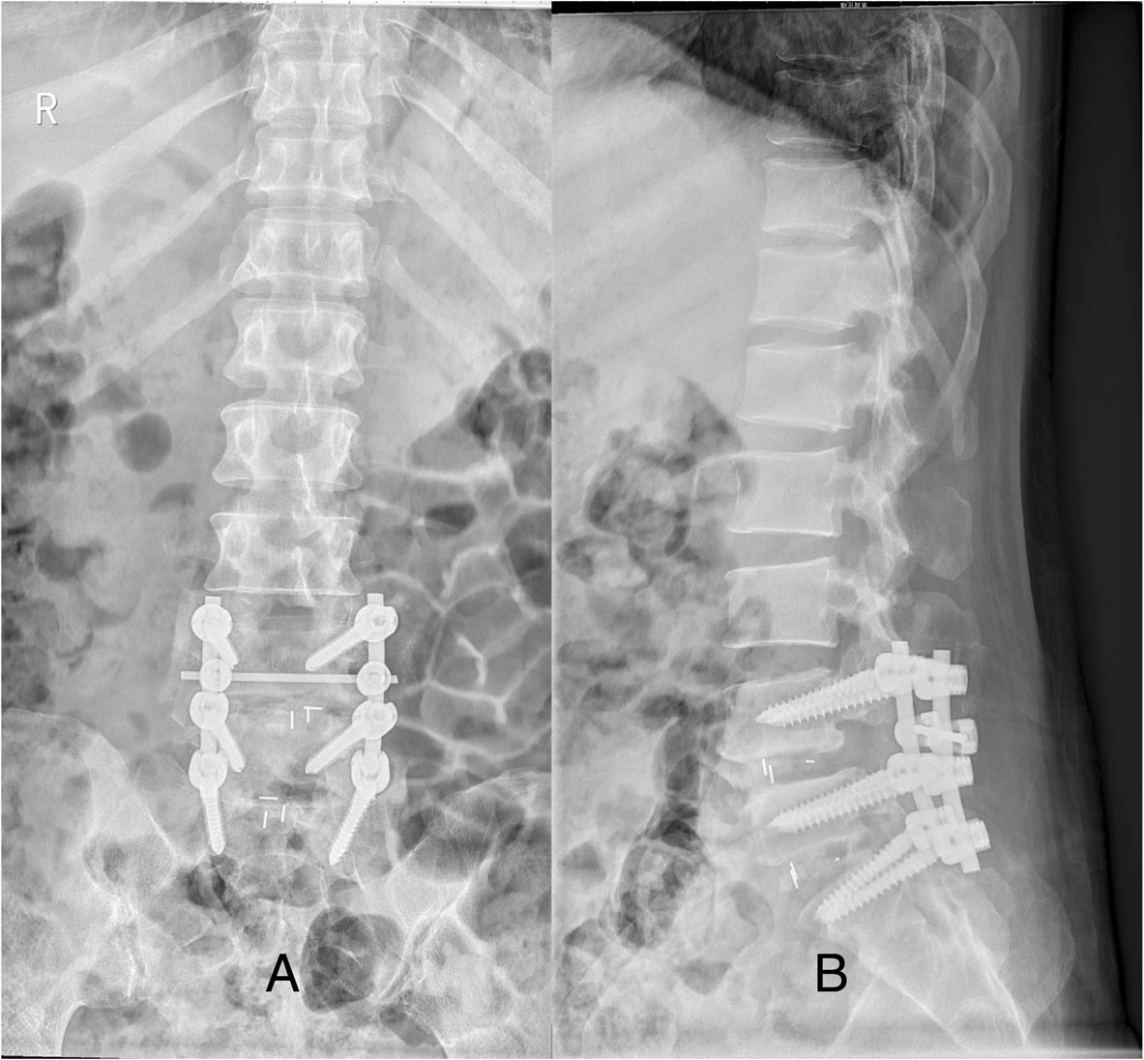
Postoperative lumbar X-ray after debridement (a and b) showed gas pseudocyst disappeared with no signs of infection
